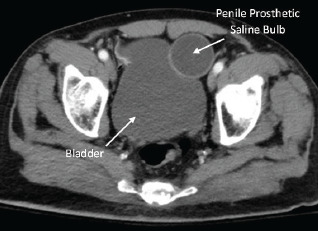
Computed tomography image of penile prosthetic saline bulb reservoir in transverse pelvic view.
